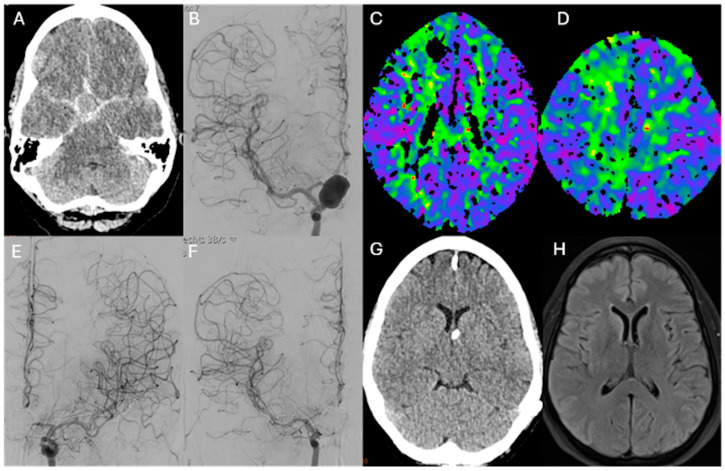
Representative case of late vasospasm. 52-year-old female patient with severe subarachnoid hemorrhage (A) due to a supraophthalmic ICA aneurysm (B) treated with stent-assisted coiling. Elevated flow velocities on transcranial Doppler (TCD) were detected on day 11, followed by CT perfusion demonstrating bilateral frontoparietal perfusion delay (C,D). Digital subtraction angiography (DSA) revealed moderate vasospasm of the bilateral middle cerebral and anterior cerebral arteries (E,F). The patient underwent three sessions of intra-arterial spasmolysis in both carotid territories. During the clinical course, no secondary infarctions developed (G,H). Note: focal parenchymal changes along the EVD catheter tract may be present and should not be interpreted as vasospasm-associated territorial ischemia.
